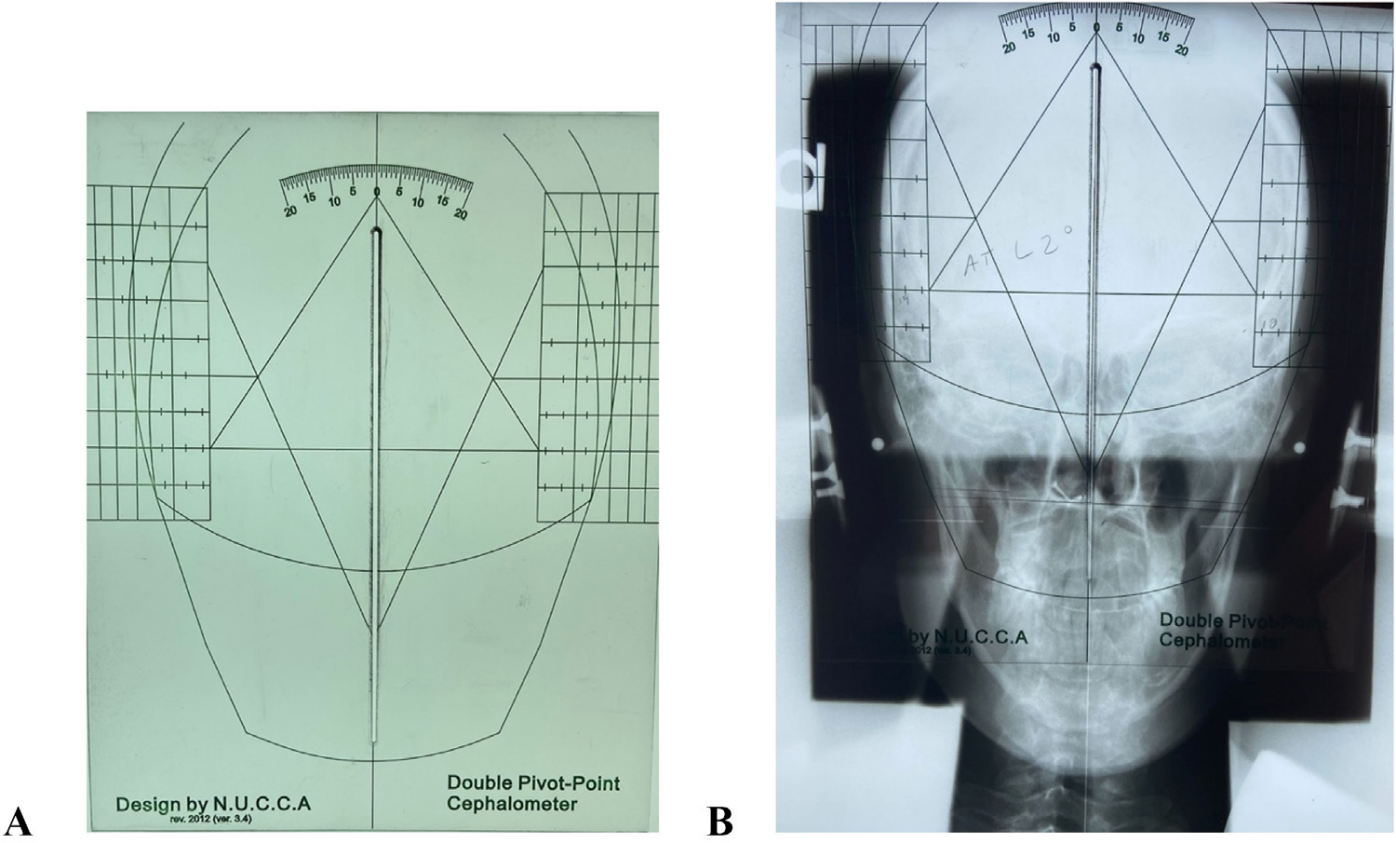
A) The NUCCA double pivot point cephalometer, which is used to divide the skull. B) An example of the cephalometer when overlaid on a plain film nasium radiograph.
